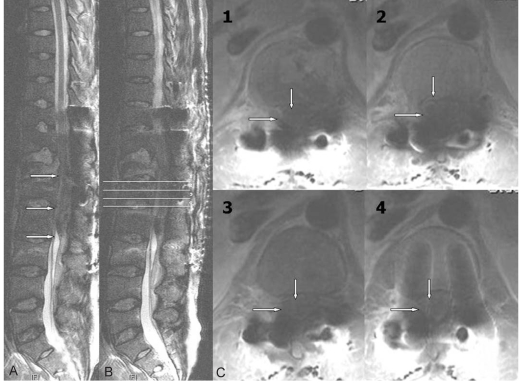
MRI with neurological deficit 7 days after the spinal trauma and fixation. Heterogeneous hypointense hematoma on the T2-weighted images was noted (A, B), and it was definitely increased on axial plane compared with initial axial images (C).
